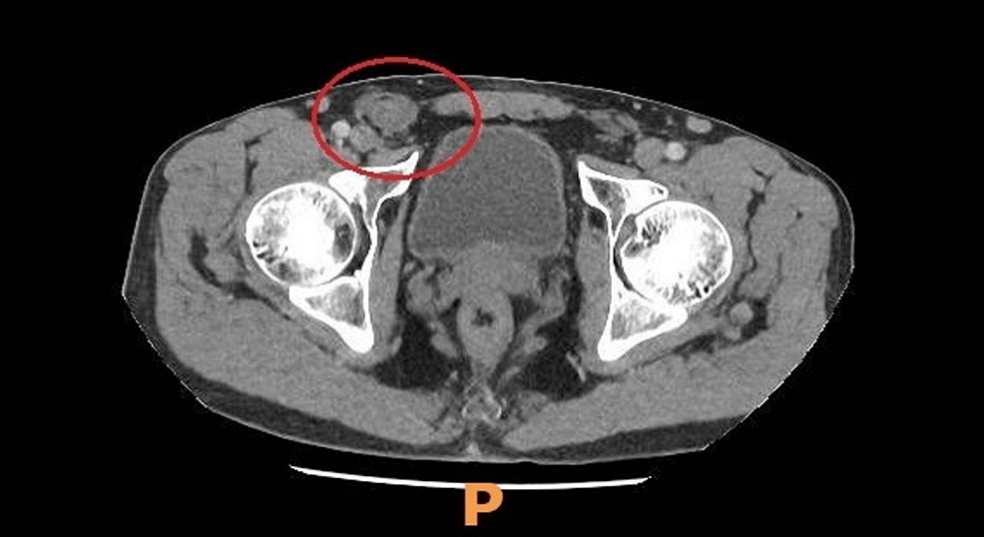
An axial view of a CT scan of the abdomen and pelvis with contrast The scan shows thickening and inflammatory changes associated with the inguinal canal likely secondary to inflammation of the contained sperma.
